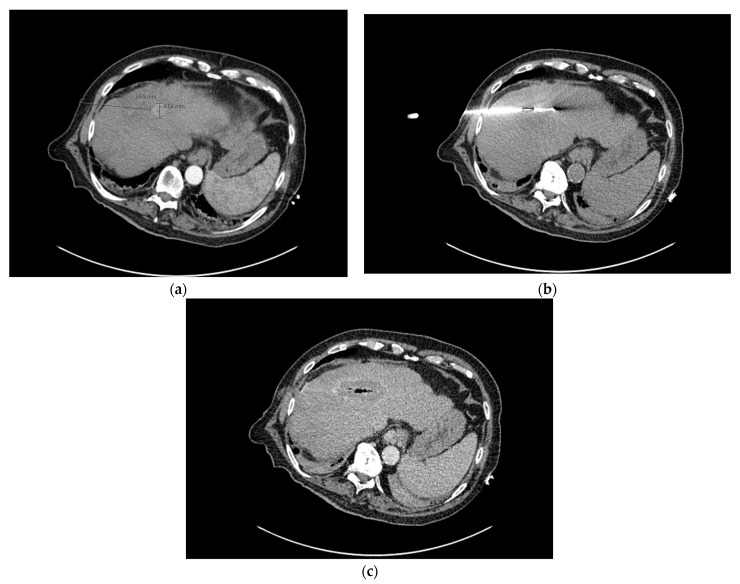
(a) Planning CT for microwave ablation of solitary hepatocellular carcinoma (Archive of Dr Fotiadis, The Royal Marsden Hospital). (b) Microwave ablation of solitary hepatocellular carcinoma (Archive of Dr Fotiadis, The Royal Marsden Hospital). (c) Post-ablation CT (Archive of Dr Fotiadis, The Royal Marsden Hospital).
